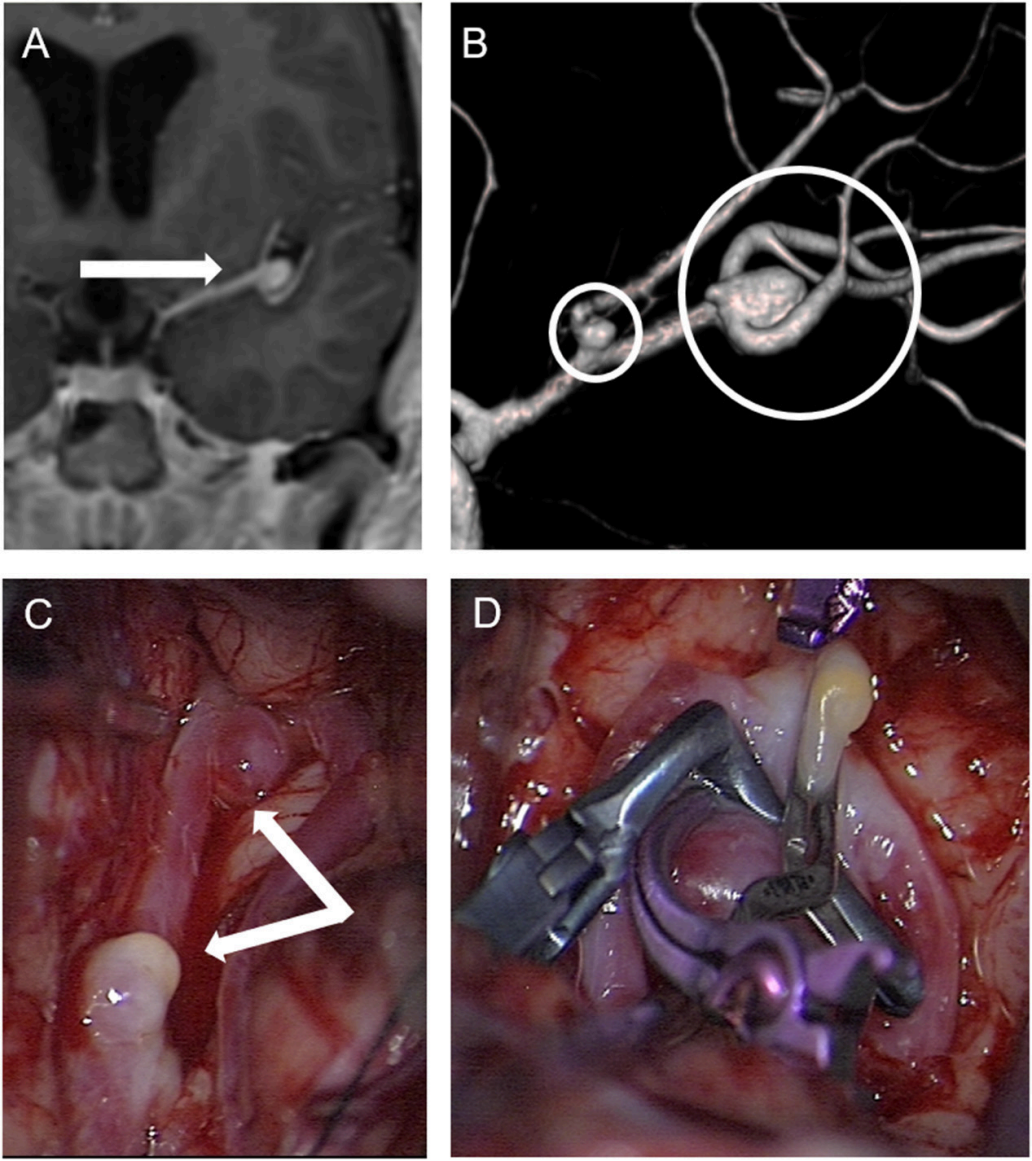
Patient No. 4 with incidental finding of two aneurysms in the left middle cerebral artery. (A) Enhanced coronal T1-weighted MRI for planning vestibular schwannoma surgery demonstrated an aneurysm in the middle cerebral artery bifurcation (arrow) and completion of cerebral angiography and three-dimensional computed angiography (B) detected another aneurysm originating from the frontal branch of the middle cerebral artery (circles) in patient No. 4. (C) Preparation of both aneurysms in the sylvian fissure of the left side via a pterional approach. and (D) microsurgical clipping.
